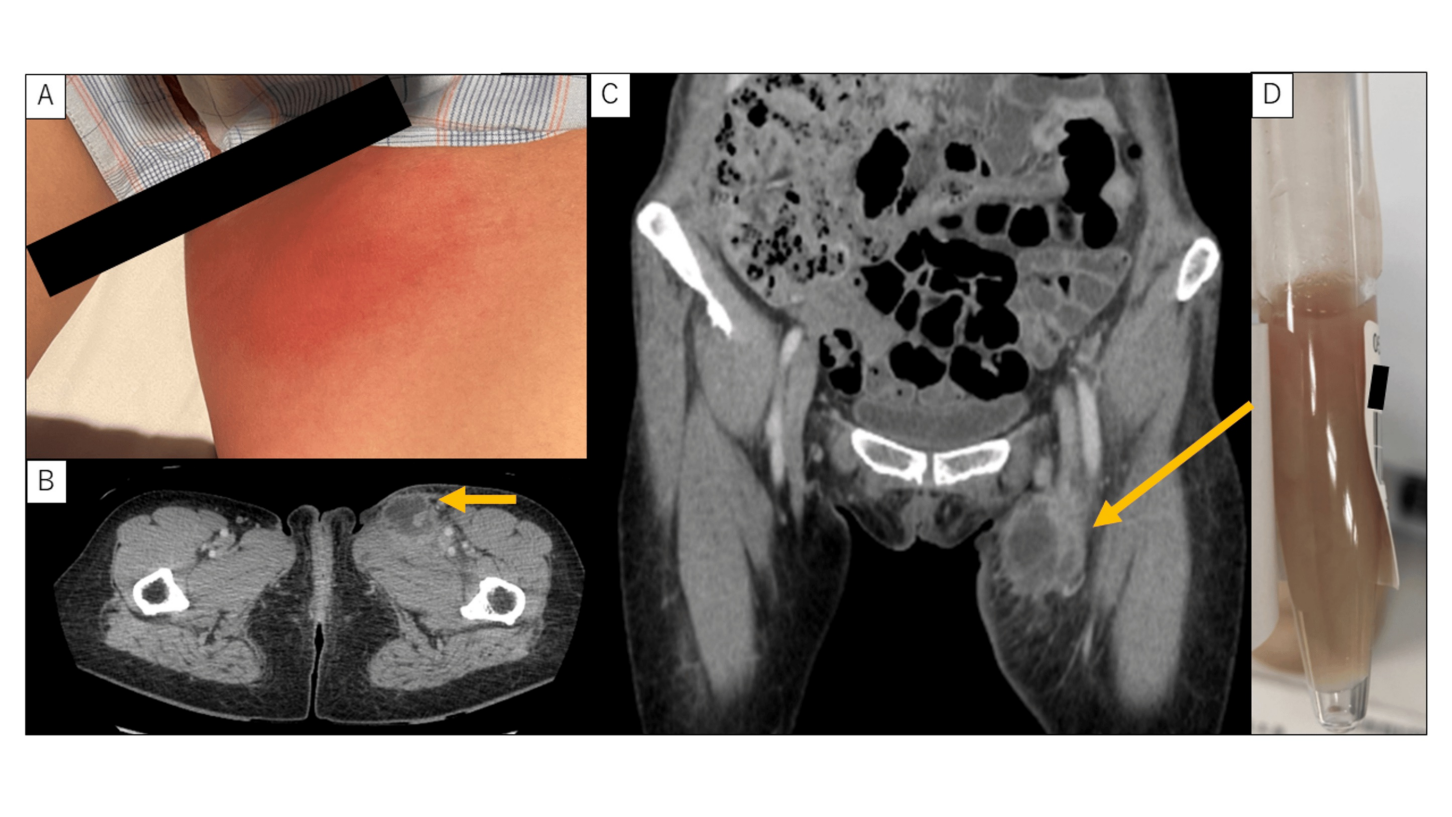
Clinical and radiologic findings of inguinal suppurative lymphadenitis (A) Gross appearance of the left inguinal region showing a fist-sized swelling. A firm, elastic lymph node was palpable with limited mobility. The overlying skin exhibited erythema and localized tenderness. (B) Contrast-enhanced sagittal computed tomography scan image of the pelvis. (C) Contrast-enhanced coronal computed tomography scan image showing an enlarged left inguinal lymph node with surrounding fat stranding. Within the node, a low-density area without contrast enhancement was observed, which is consistent with abscess formation. (D) Image of the purulent aspirate obtained via drainage of the left inguinal abscess. Approximately 8 mL of whitish, turbid pus was collected.
